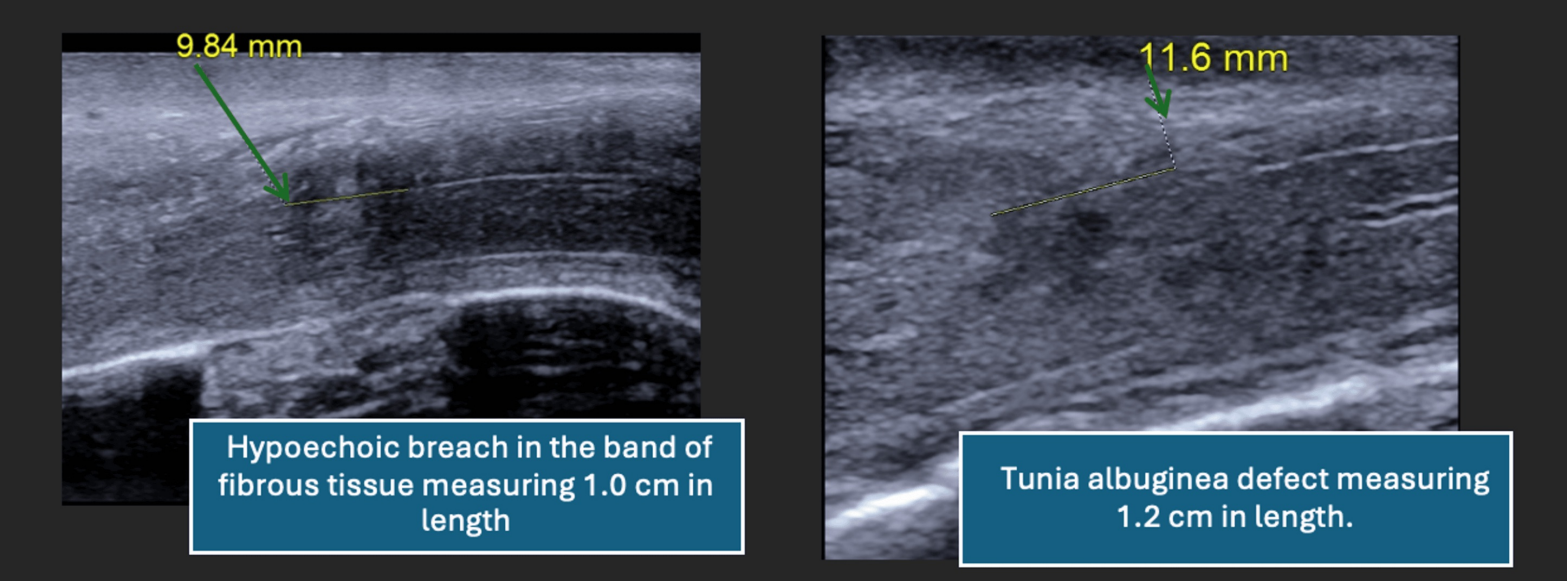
Ultrasound images of tunica albuginea tear. Longitudinal ultrasound views of the left corpus cavernosum demonstrate a hypoechoic defect measuring approximately 1.0 cm (left panel) and 1.2 cm (right panel), consistent with a tunical breach. No hematoma is visualized. The hyperechoic surrounding tissue suggests edema. Arrows denote the site of the tunica albuginea disruption.
